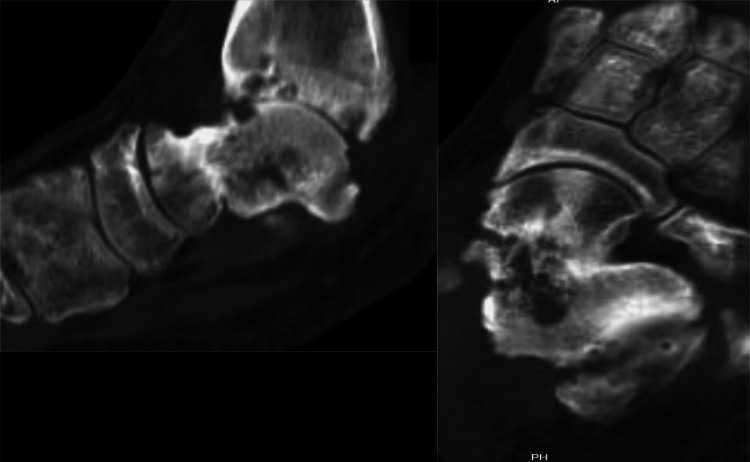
Sagittal and axial views of the CT scan of the left ankle
